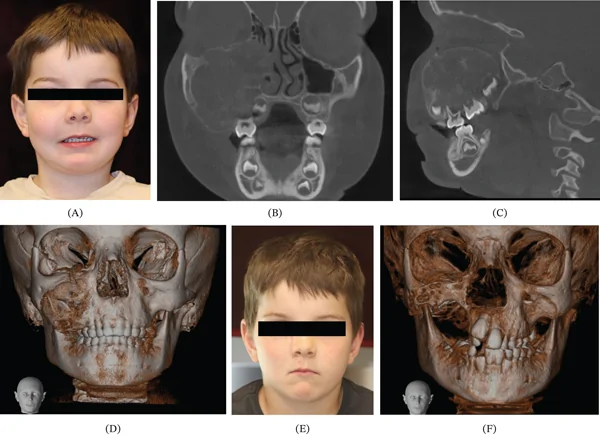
(A) Preoperative frontal repose photograph showing swelling of the right cheek. (B) Preoperative CT (coronal) imaging of facial bones with contrast showing a 4.7 × 3.9 × 3.5 cm expansile, well delineated lesion arising from the right maxilla with internal groundglass attenuation. (C) Preoperative CT imaging (sagittal) imaging. (D) Preoperative 3D reconstruction. (E) Postoperative frontal repose photograph showing normal facial expression without facial swelling and linear scar at the site of surgical intervention at 12 months. (F) Postoperative 3D reconstruction status post right total maxillectomy and post embolization of right internal maxillary artery showing no evidence of the previously seen right maxillary lesion through 16 months of follow‐up; the remaining paranasal sinuses are clear.
